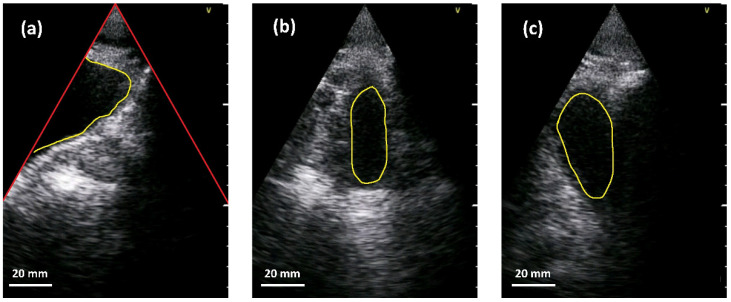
Examples of bladder segmentation (yellow). Red lines in the left sub-image indicate the borders of the ultrasound fan above which there is no valid information. (a) Partially visible bladder. (b) Almost empty bladder near the center of the US-fan. (c) Additional dark regions due to pubic bone shadow or colon.
